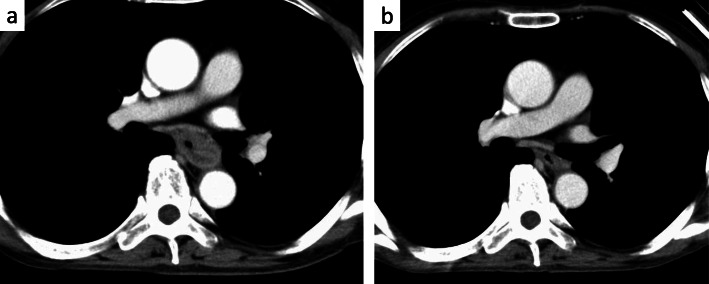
Chest CT scan showing a the esophageal cancer contacts to the left main bronchus and both the lower lobe of the lung before NAC. b After neoadjuvant chemotherapy, esophagus wall thickening had somewhat improved. However, invasion of adjacent organs could not be ruled out
